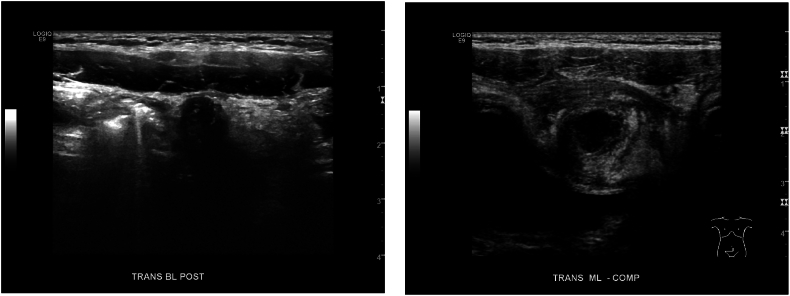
Pre-operative ultrasound.
